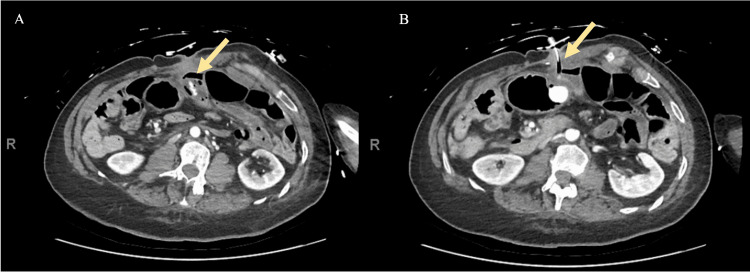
(A) Computed tomography angiography during the arterial phase demonstrating intraluminal contrast extravasation in a small bowel loop with associated mild mural edema and fat stranding (yellow arrow), suggesting this to be the source of the GI bleeding. (B) Delayed phase imaging demonstrating layering intraluminal contrast with concern that the feeding tube was partially extending through a portion of the small bowel wall (yellow arrow).
